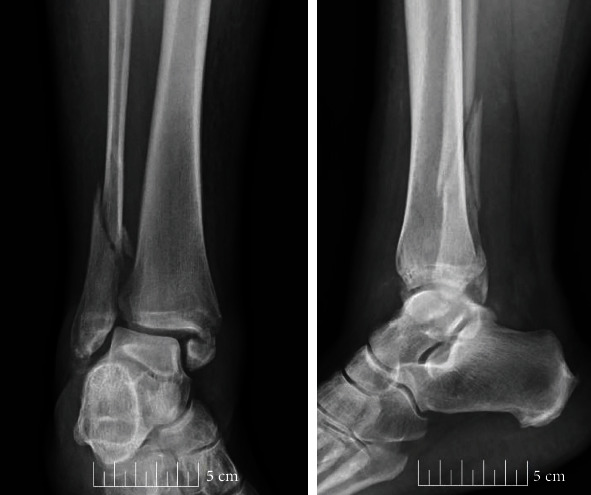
Initial radiographs demonstrating trimalleolar ankle fracture.
